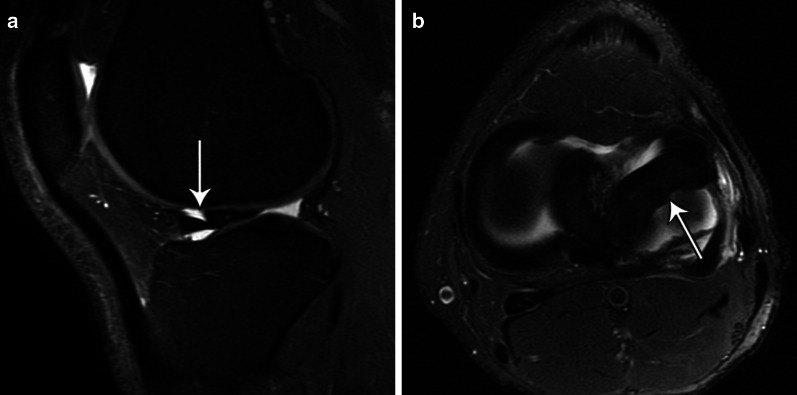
Sagittal T2-weighted fat-suppressed MR image (a) shows a double delta sign (thin arrow), typical for flipped meniscus. Axial image (b) shows anterior dislocation of the posterior horn of lateral meniscus (thin arrow)
